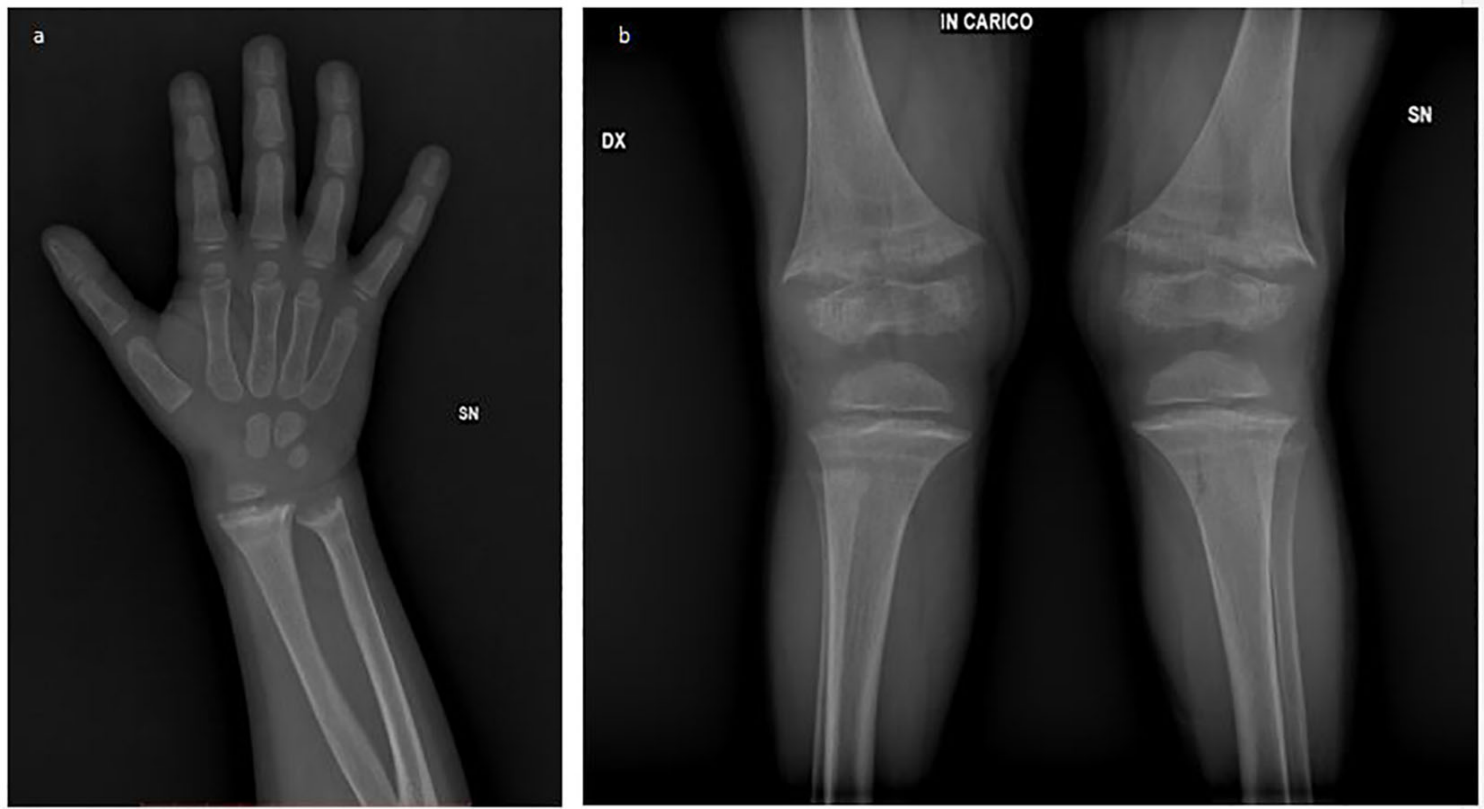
Radiographic features of rickets: (a) The rachitic wrist showing metaphyseal widening, irregular splaying, cortical thinning, and delayed ossification. (b) Bowing of long bones with genu valgus deformity and cup- or bell-shaped metaphyses.
